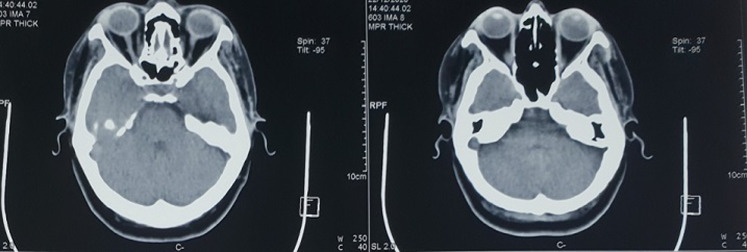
control computed tomography scan performed two years later was normal without mass
